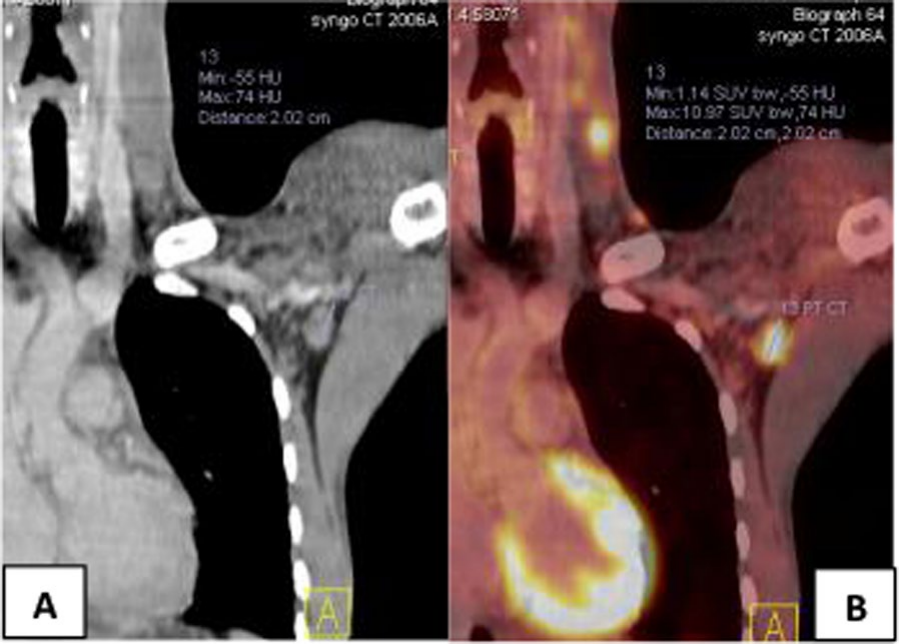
A 42-year-old male patient with NHL, treated with anti-cd20 antibody rituximab 10 months prior to vaccination. After 1 year of disease-free, PET/CT reported new left axillary, supraclavicular and lower cervical LNs. Clinical data revealed that he received the 2nd dose of Pfizer-BioNTech vaccine in his left arm 6 days prior to PET/CT. A Coronal CECT and B coronal PET/CT images revealed enlarged FDG-avid left axillary, supraclavicular and lower cervical LNs, largest measures 2 cm in left axilla of 11 SUVmax
